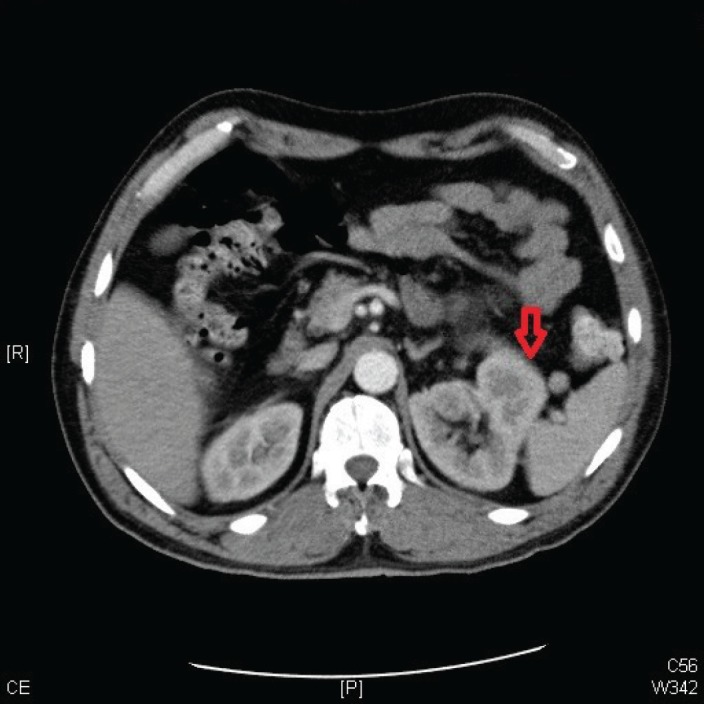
A CT scan of the abdomen and pelvis showing a left renal mass 13 cm in diameter with features suggestive of primary RCC.
